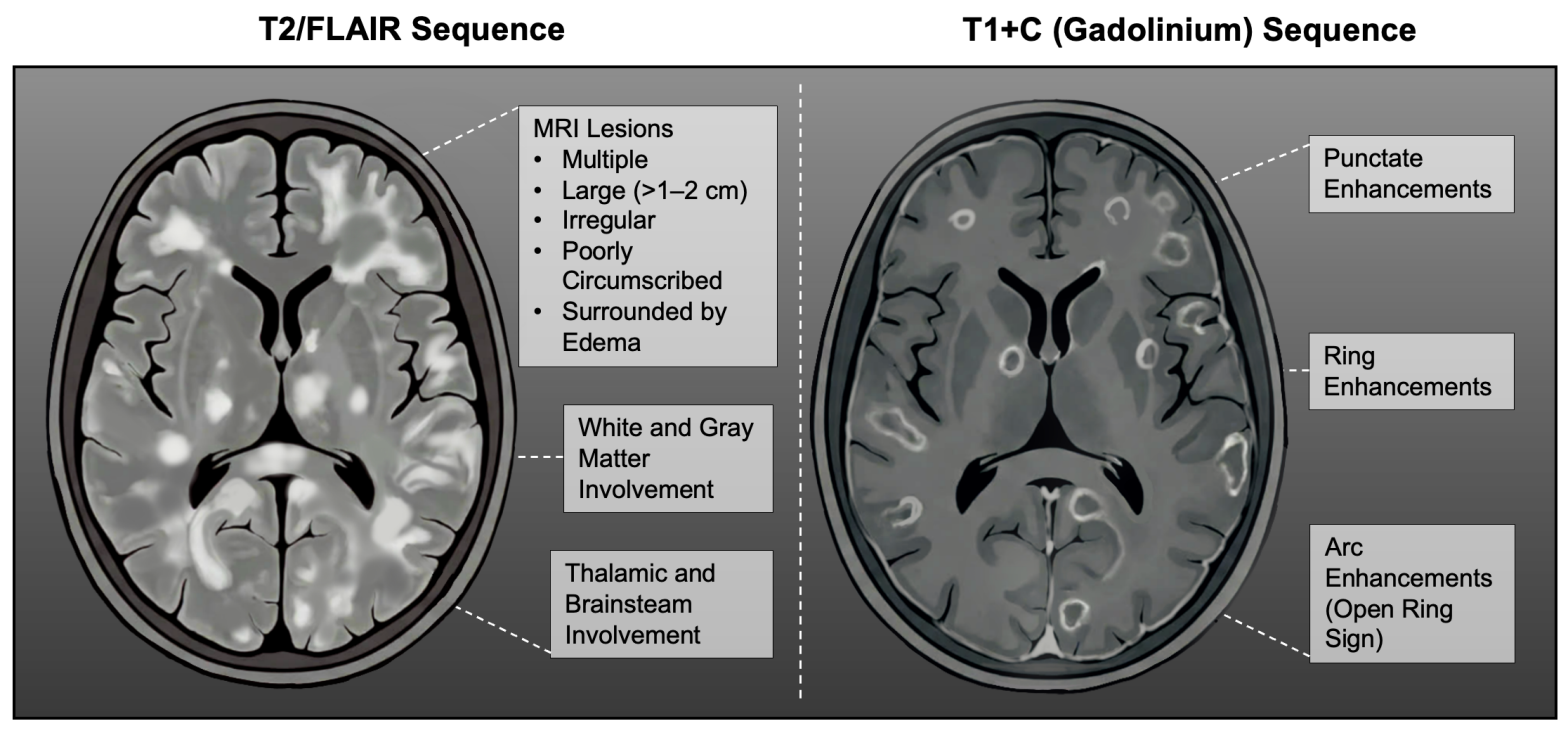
Typical MRI changes in ADEM visible in T2/FLAIR and T1+C (Gadolinium) sequences.
